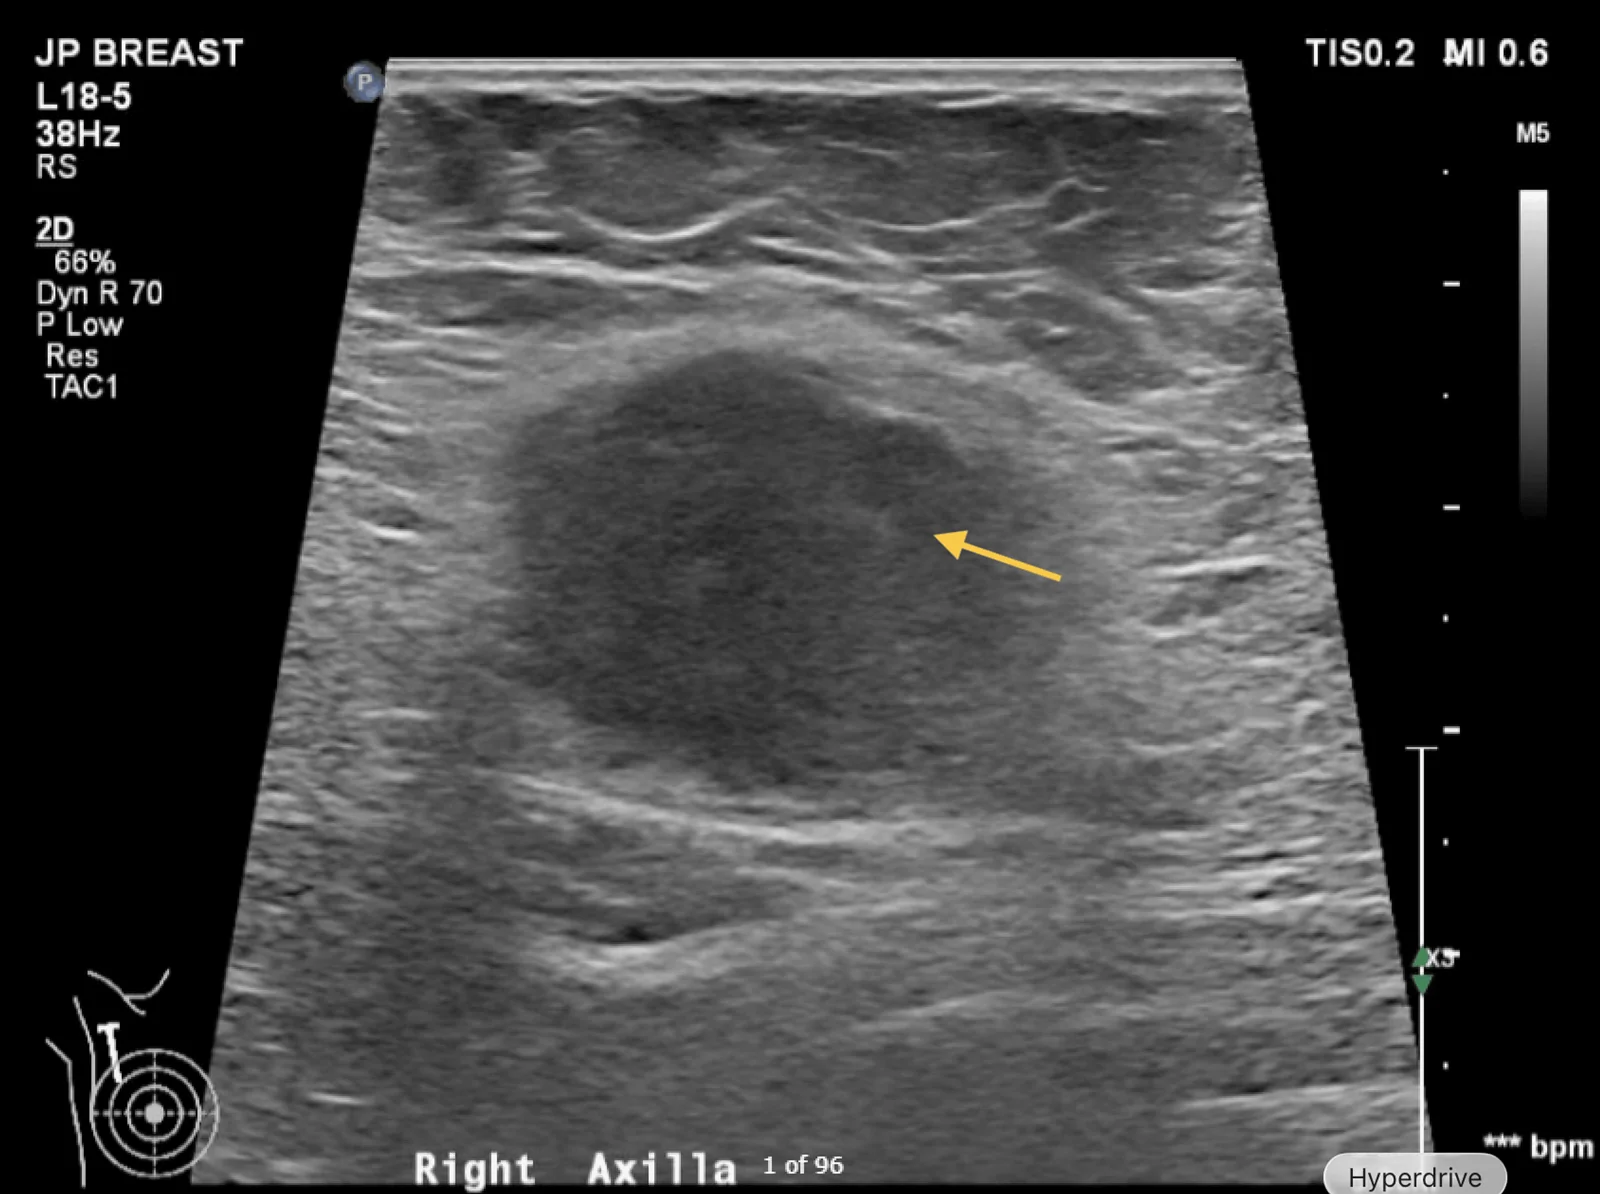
Targeted ultrasound demonstrating a suspicious right axillary lesion (arrow).
